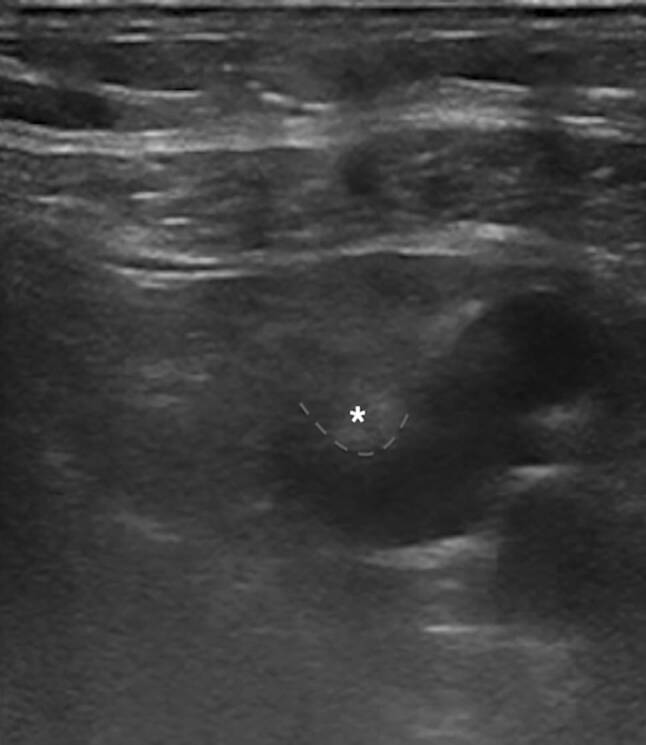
Ultrasound-guided direct access by direct indentation of the axillary vein (*)
